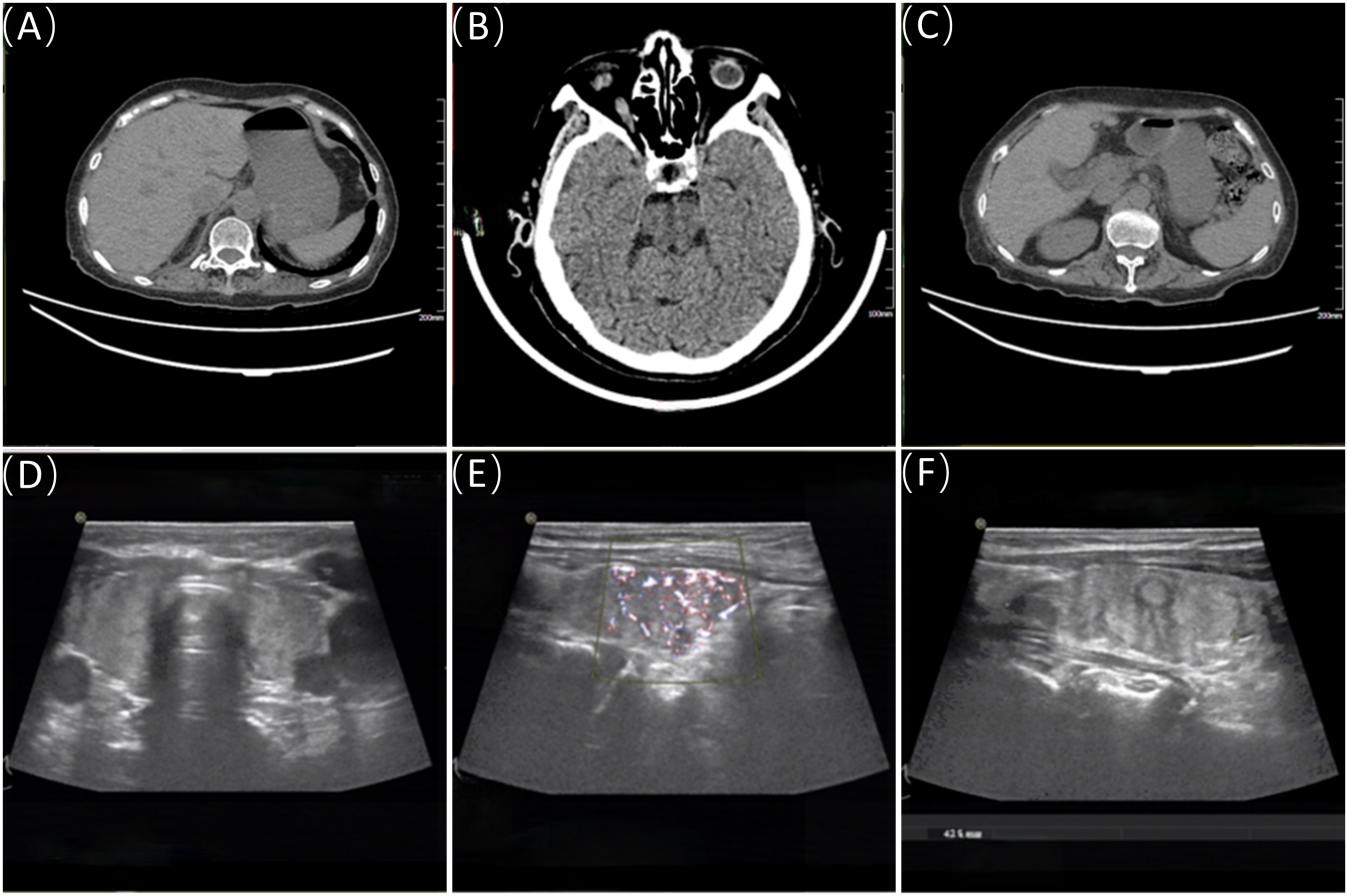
Adrenal CT, pituitary CT, and thyroid ultrasound. (A, C) show the CT images of the right and left adrenal glands, respectively; (B) displays the pituitary CT image. (D–F) are ultrasound images of the thyroid.
